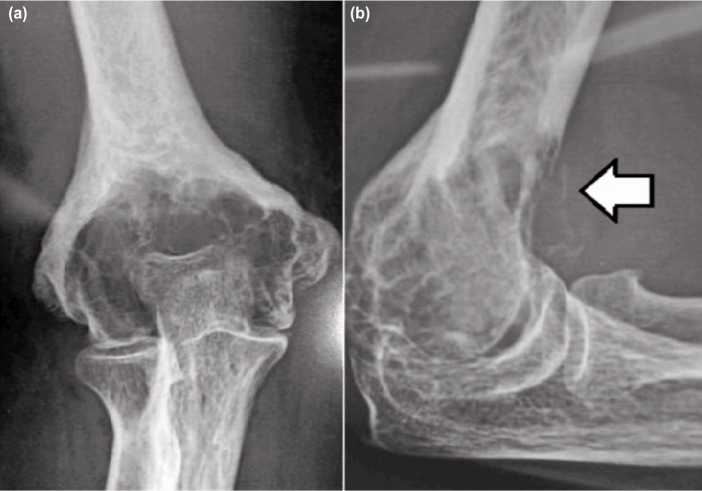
(a) Pre-operative plain radiograph of the elbow-AP showing distal humeral lytic lesion and (b) Lateral radiograph showing breach in anterior cortex (arrow).
